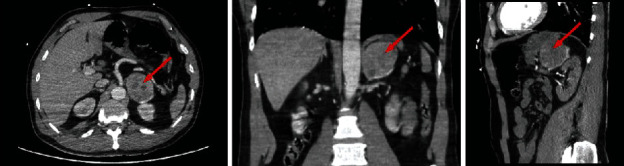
Abdominal computed tomography scan showed a left-sided adrenal mass of 5.7 cm (arrow).
